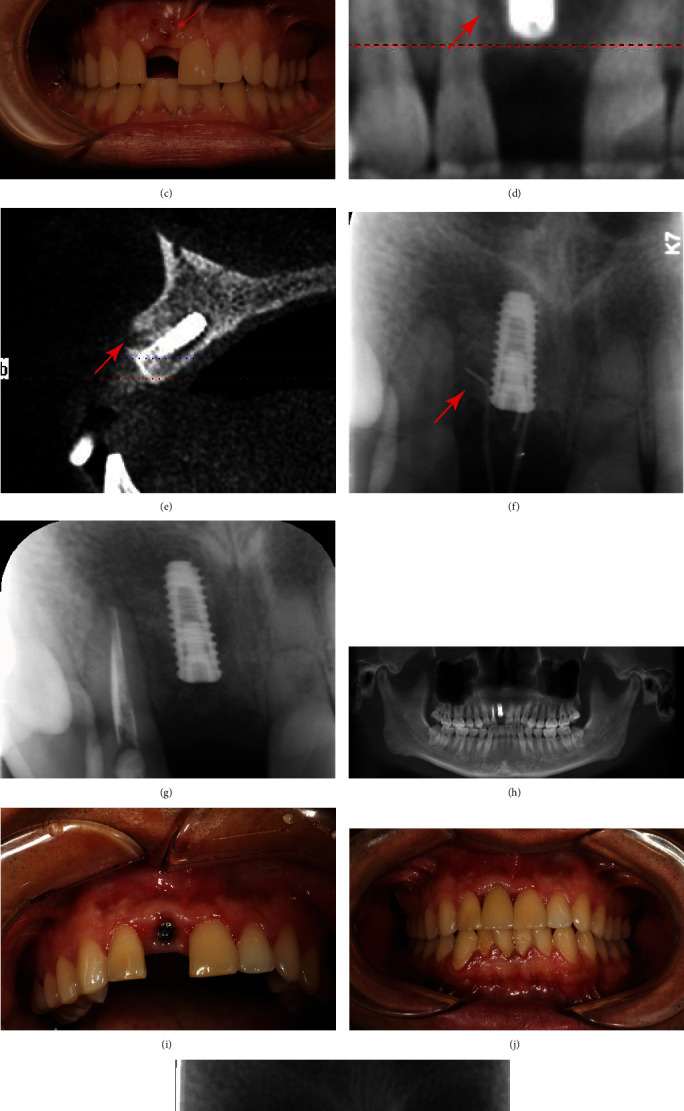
Case 2: (a) Preliminary Cone-beam CT before implant placement. (b) A coronal image of site #11 indicated the absence of labial bone and poor socket healing. (c) Fistulas were observed in the labial mucosa around implant #11(red arrow). (d, e) Follow-up visit after implant placement for 5 months, radiography appeared to indicate a mesial spread of apical periodontitis in tooth #12 to the coronal peri-implant defect (red arrow). (f) Apical radiograph revealed a diagnostic Gutta-percha cone tracing from mucosal fistulas to tooth #12 (red arrow). (g) Periapical radiographs at 1-month follow-up after filling the root canal with Vitapex. (h) Cone-beam CT indicated that RCT of tooth #12 was suitable, and the peri-implant bone defect was healed after half a year. (i) Intraoral photographs half a year after root canal filling. (j) The intraoral photograph of the implant-supported restoration 7 months after RCT showed healthy peri-implant mucosa. (k) Apical radiograph showed no defects of peri-implant supporting bone. Root canal therapy, RCT.
